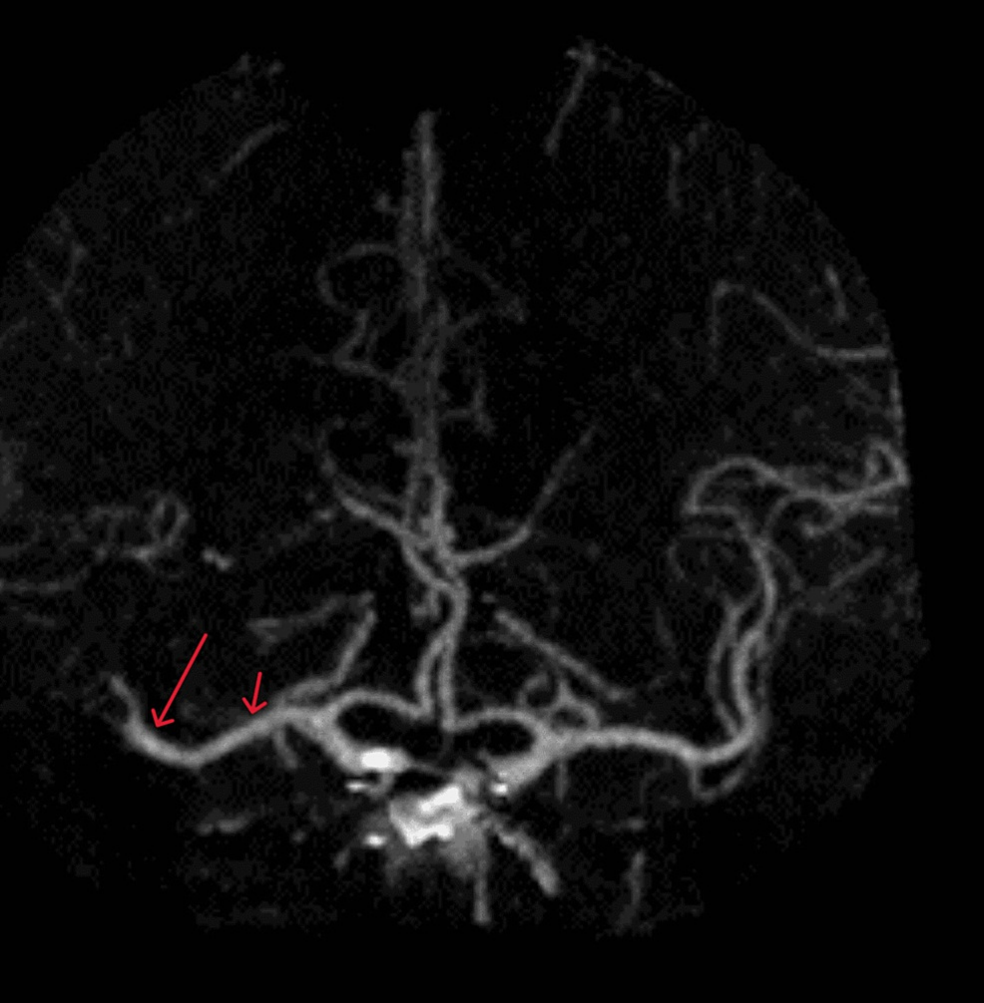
CT arteriography showing M2 occlusion (long red arrow) and lenticulostriate patency (short red arrow)
